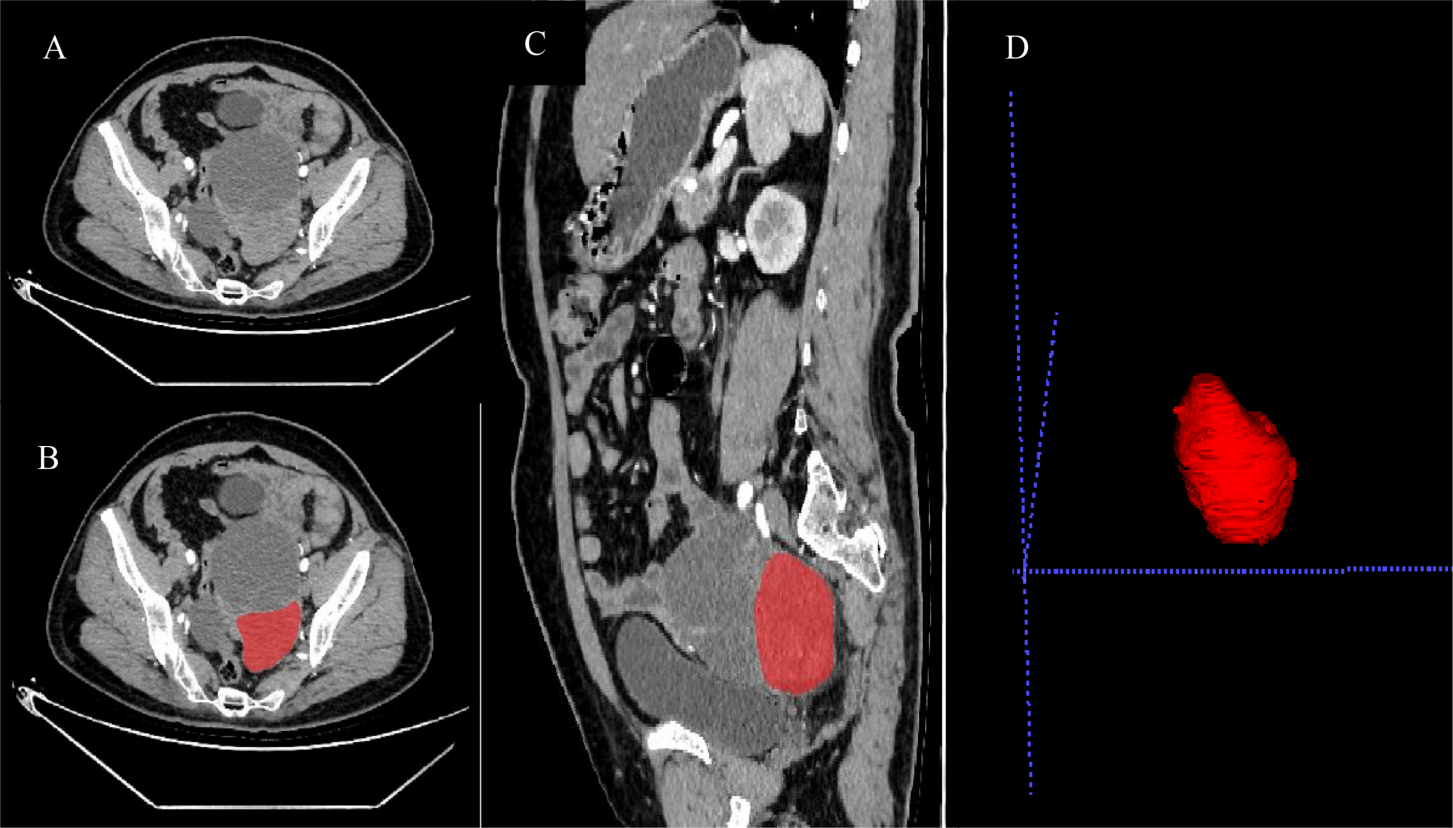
Preoperative enhanced CT arterial phase images of patients with wild-type BRCA (A), as well as processing images (B–D) after ROIs were drawn by ITK-SNAP software.
